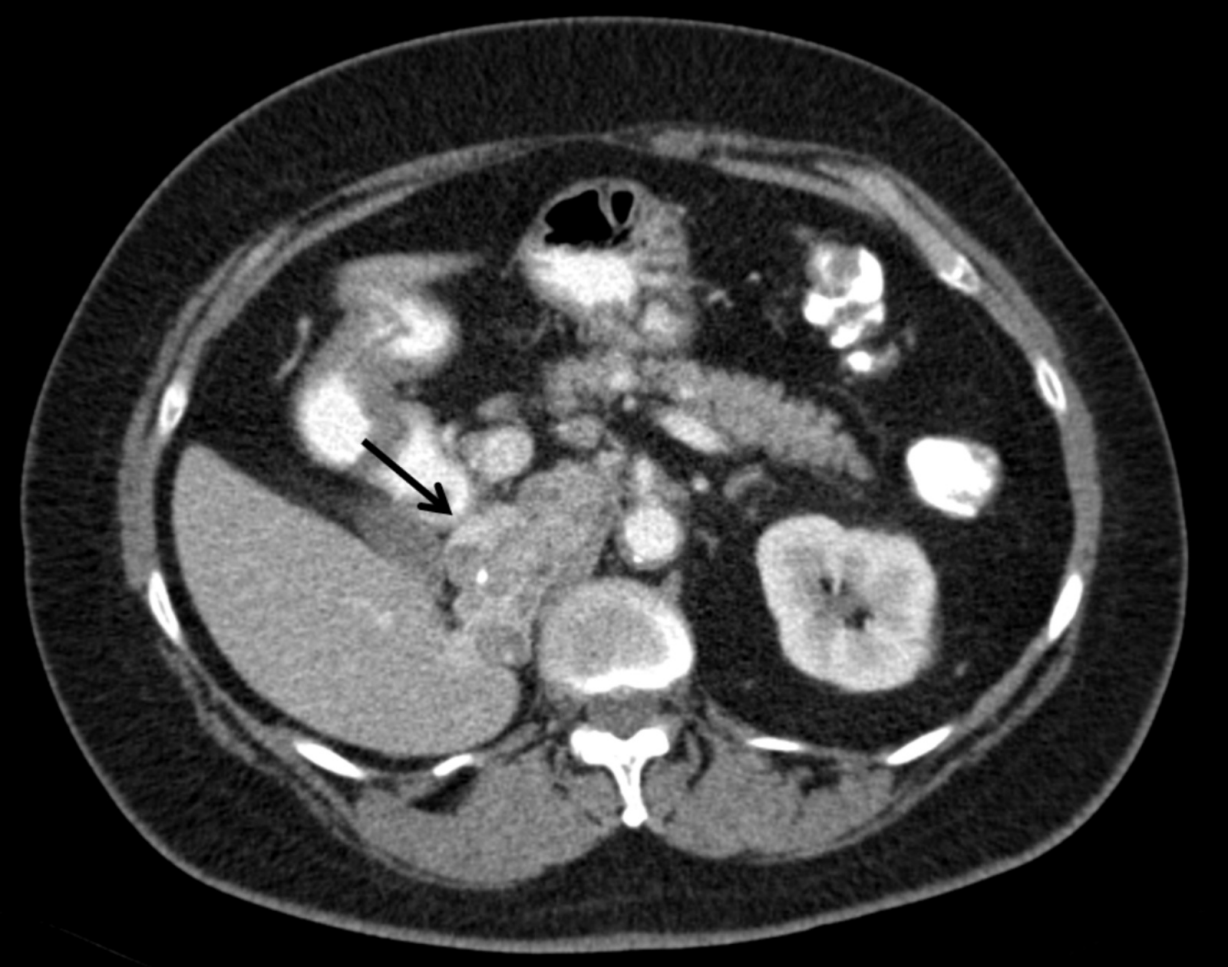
Transverse plane of computed tomography scan revealing invasive pheochromocytoma (black arrow)
